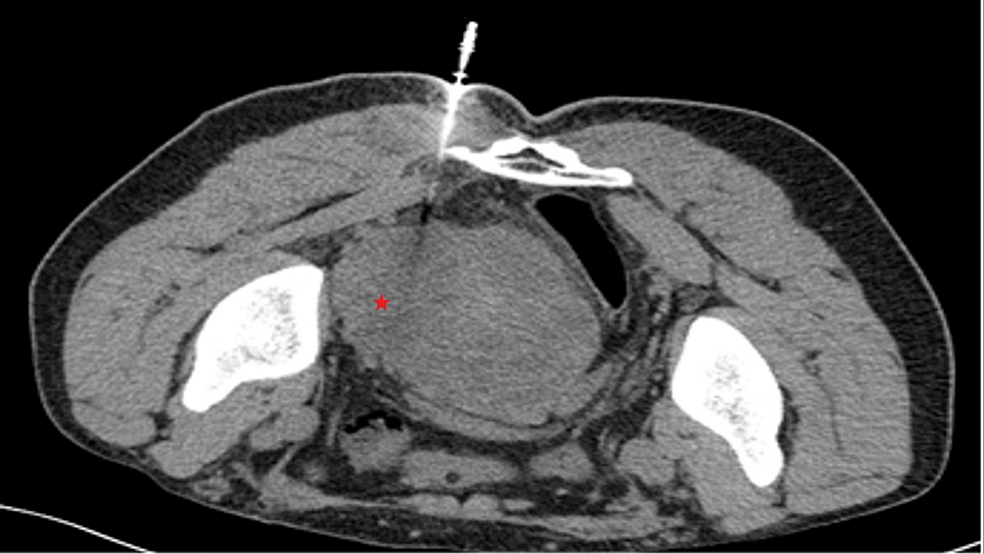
CT-guided core biopsy (Tru-cut), with the red asterisk showing the location of the tumor.
